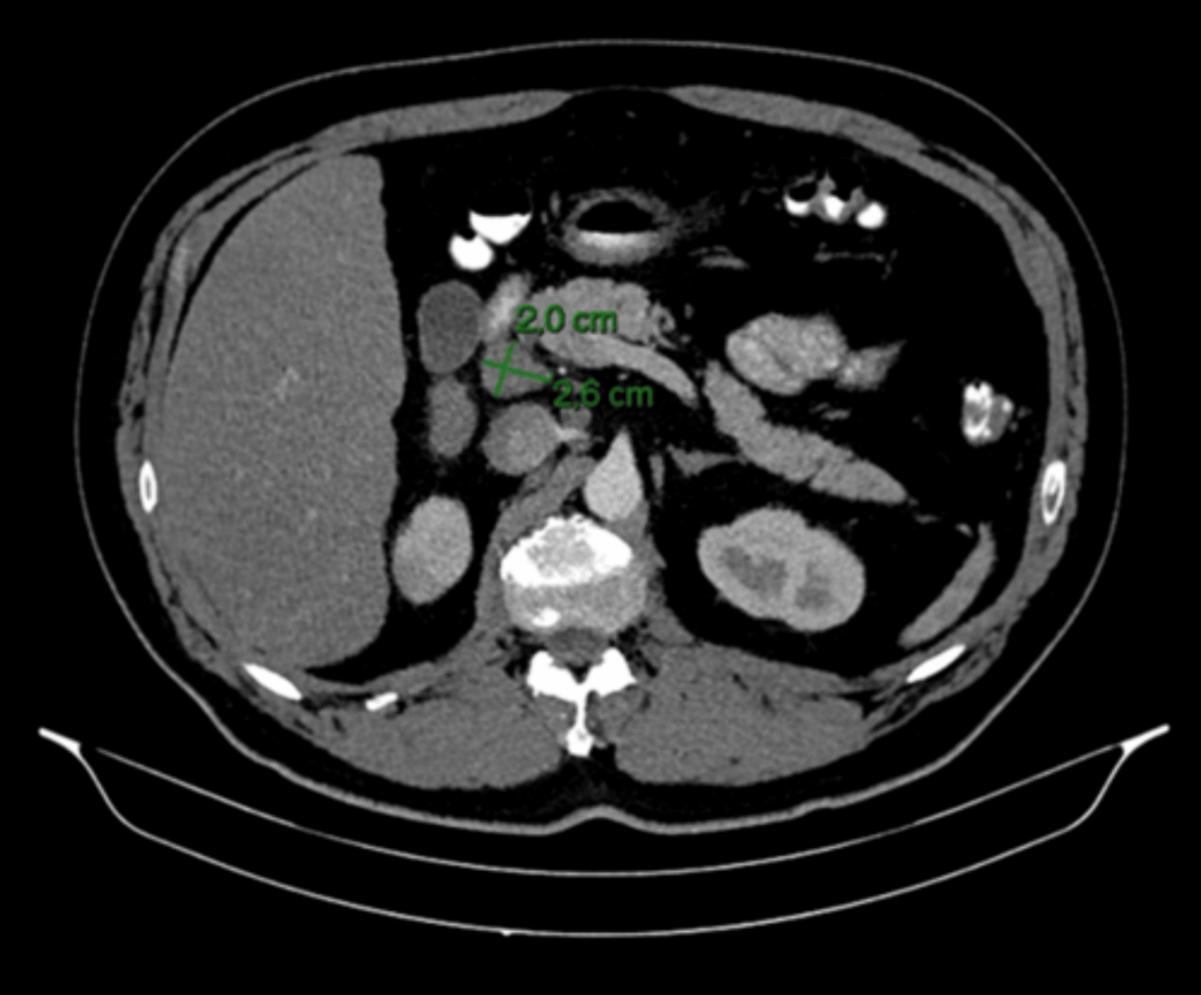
CT abdomen (axial view), showing enlarged lymph node adjacent to the pancreatic head (2.6 × 2.0 cm) in the right upper quadrant
